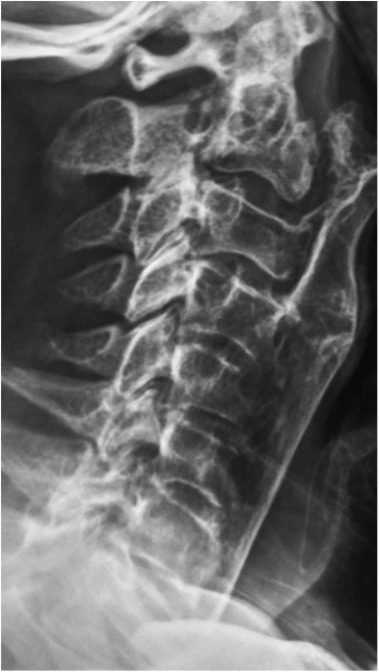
Lateral C-spine radiograph. There is extensive bulky calcification of the anterior longitudinal ligament (ALL) from C2 level extending to the upper thoracic spine, affecting more than four contiguous vertebrae. The lesion impinges upon the upper airways. There is no evidence of calcification of the posterior longitudinal ligament. The facet joints and disk spaces are preserved.
